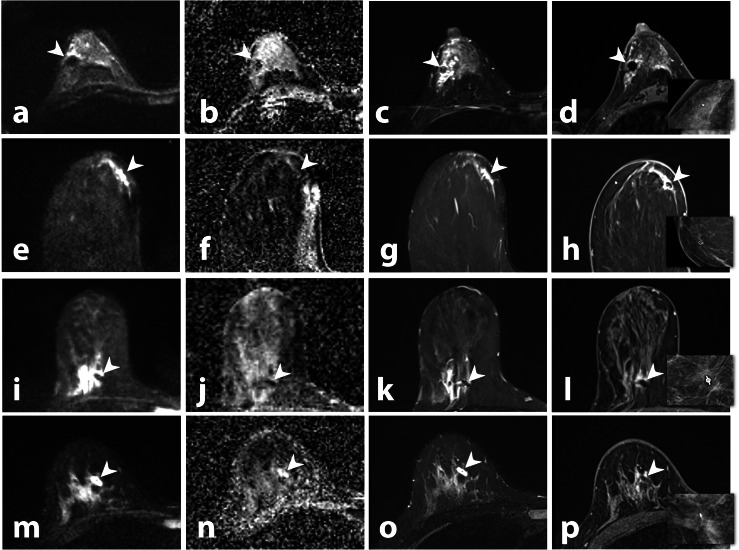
Clip artifact examples (white arrowheads) on diffusion-weighted images (*b* = 800 s/mm^2^) (1st column); ADC maps (2nd column). T2-weighted turbo inversion-recovery magnitude images (3rd column), and contrast-enhanced fast-suppressed T1_Flash3D images (4th column) for Bard-UC (a–d), Somatex-TP (e–h), Somatex-TV (i–l), and devicor mammatome hydromark (m–p). Small inserts depict mammographic post-biopsy clip control findings (lower right angle in the 4th column)
